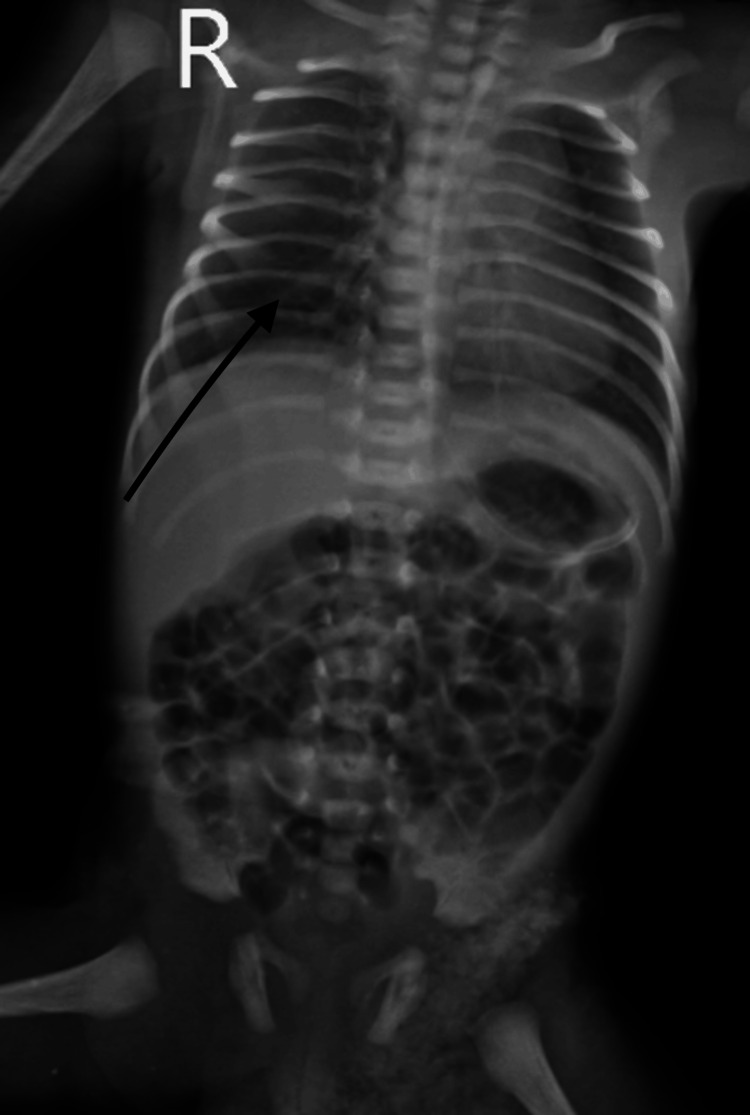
Chest X-ray X-ray showing diffuse, bilateral, and symmetrical granular opacities with bell-shaped thorax and air bronchograms is evident
